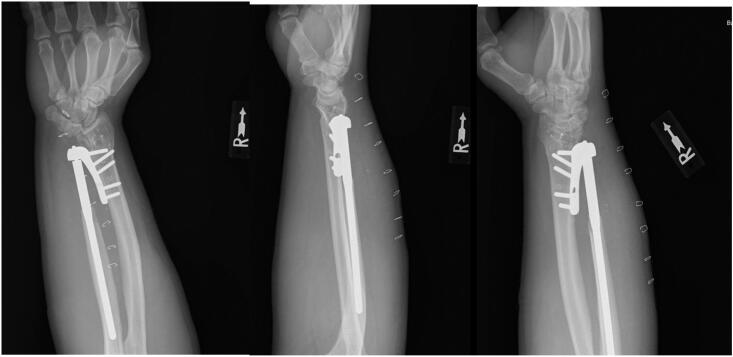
Anteroposterior (AP), lateral, and oblique 2 week post-operative radiographs showing the Scheker prosthesis. The site of the DRUJ osteotomy is seen.
